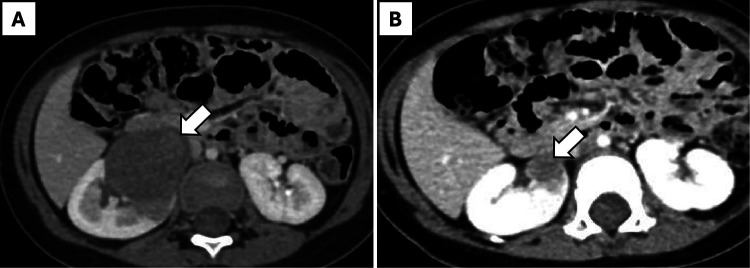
A 12-month-old girl with WAGR syndrome and right-sided Wilms' tumor. (A) Pre-chemotherapy axial CT scan showing a large renal mass (arrow). (B) Post-chemotherapy axial CT scan demonstrating regression of the mass (arrow).
